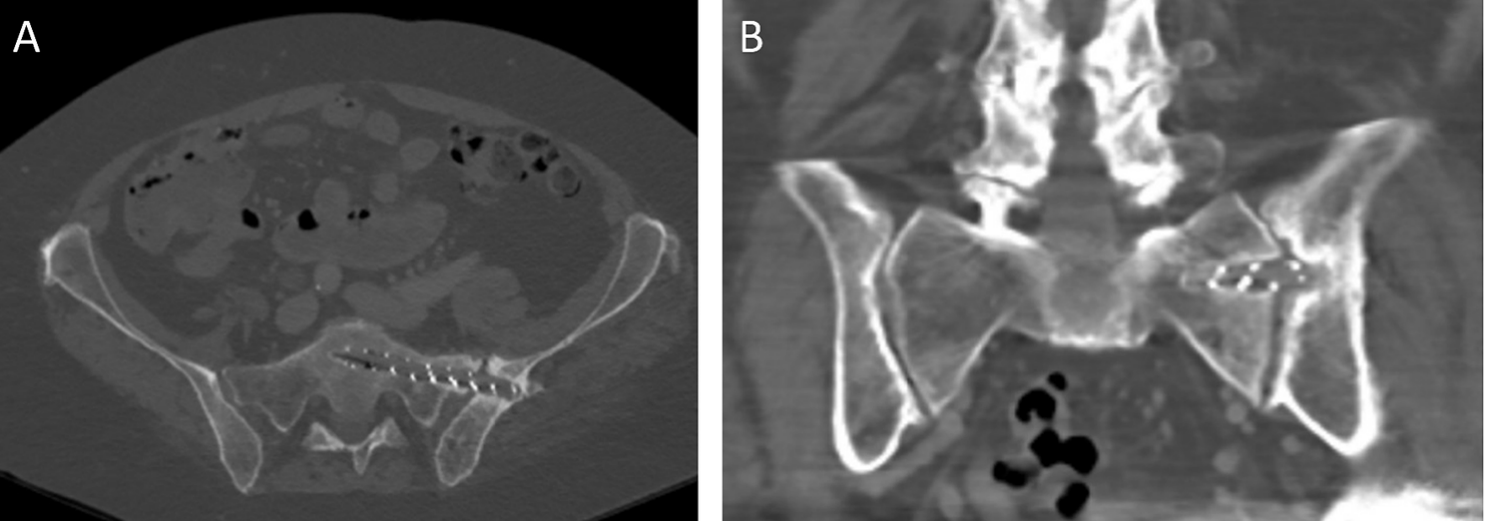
Post-operative CT scan showing adequate positioning of the photodynamic nail from the left iliac bone into the S1 vertebral body on (A) axial and (B) coronal planes
